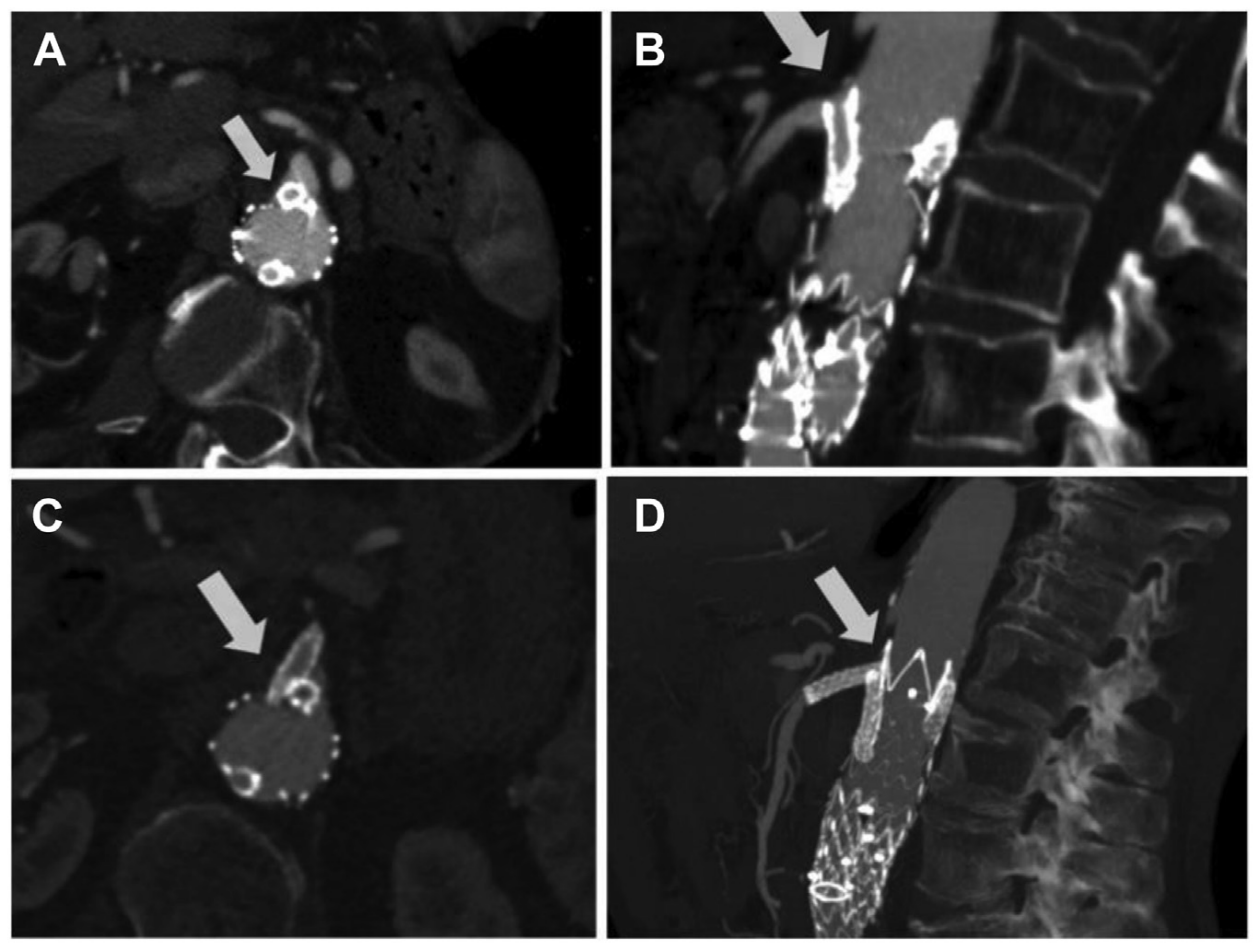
A,B, Axial and sagittal preoperative computed tomography angiography (CTA) views showing partial coverage of the superior mesenteric artery (SMA) ostium by the right renal artery (RRA) chimney graft. C,D, Axial and sagittal postoperative CTA views showing the stent positioned in the SMA.
